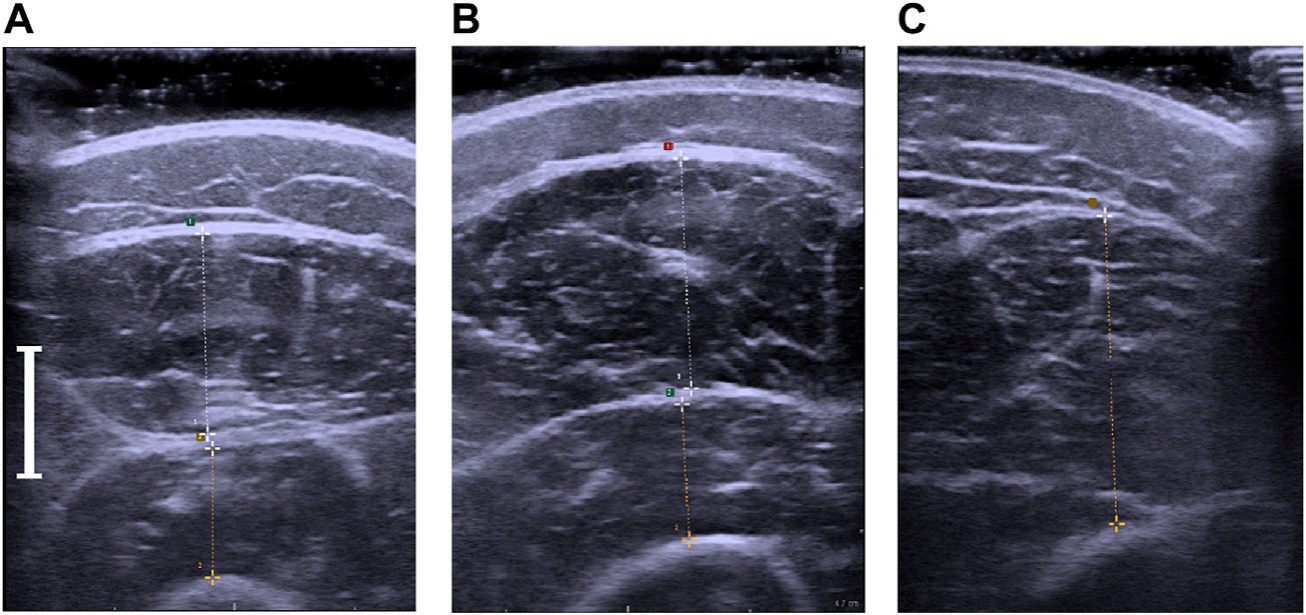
(A). Ultrasonographic measurement of the m. rectus femoris and m. vastus intermedius. (B) Ultrasonographic measurement of the m. vastus lateralis and m. vastus intermedius. (C) Ultrasonographic measurement of the m. vastus medialis. The white indicator shows the length of 1 cm.
